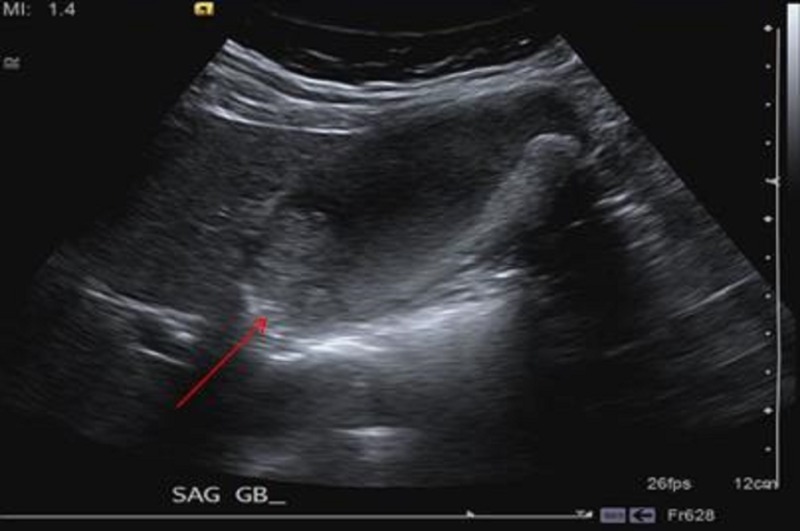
Initial right upper quadrant ultrasound. The image demonstrates intraluminal hypoechoic (red arrow) material. Given the appearance and lack of discrete internal flow, it appears to be most likely due to gallstones or tumefactive sludge, which is a benign mimic of a neoplasm.
